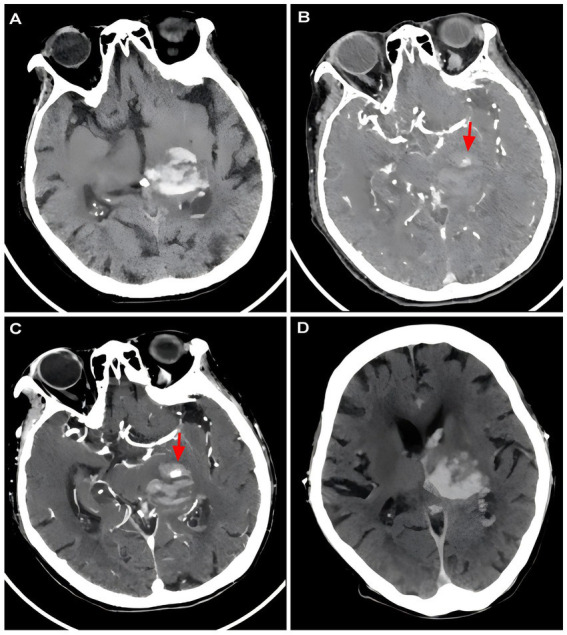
A 75-year-old woman with a left thalamic hemorrhage. The onset time was 13:00 on 30 April, and the time of arrival at the emergency department of the hospital was 14:20. (A,B) First CT and enhanced CT at 14:36, a hematoma volume was 12.90 mL, spot sign positive (red arrow); (C) sequential CT scan at 14:40 with brush sign positive and isolated morphology (red arrow); and (D) re-examined CT at 18:24, and the hematoma volume was 13.55 mL without significant hematoma expansion.
